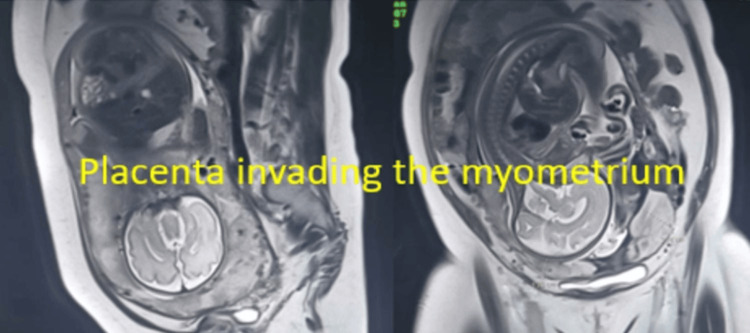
Anterior adherent placenta previa on MRI
